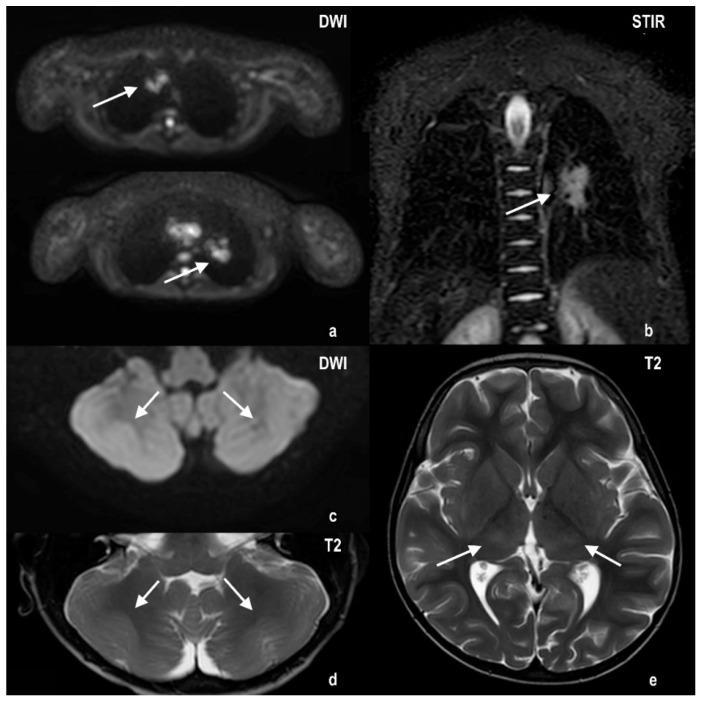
Thoracic MRI shows DWI restriction areas corresponding to the right paratracheal lymph node chains, associated with a left peribronchial area presenting a similar aspect (panel (a): axial DWI; panel (b): coronal STIR). Cerebral MRI shows bilateral symmetric cerebellar cortex swelling and T2 hyperintensity, with DWI (b1000). Restricted diffusion from cytotoxic vasogenic edema can be seen (panels (c,d): cerebellar DWI and T2, respectively), along with T2 hyperintensity of posterior thalami (panel (e): axial T2).
